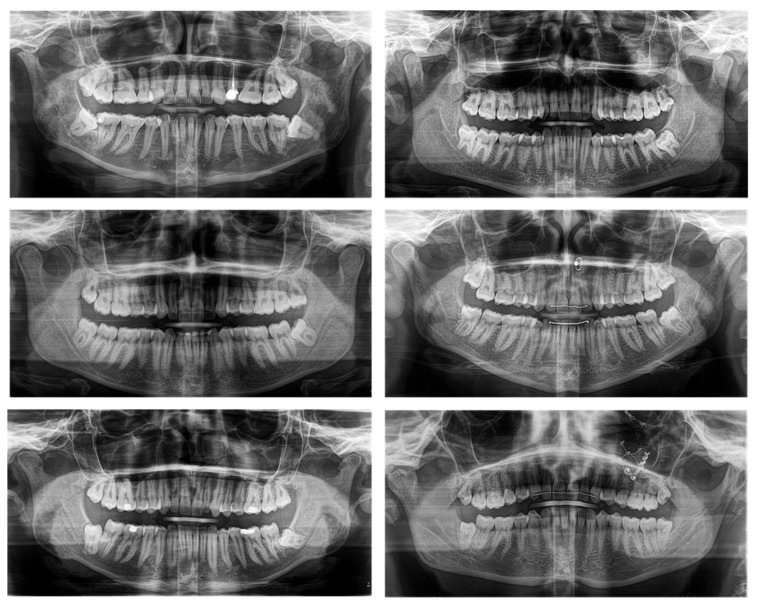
Study participants’ two-dimensional panoramic radiography (PAN) providing anatomical information about the angulation and position of the mandibular third molars (MTM) and the spatial proximity between the roots of the MTMs and the mandibular canal (MC). All participants showed an indication for the removal of MTMs and a close relationship between the MC and the MTM; therefore, these were considered high-risk cases and show indication for three-dimensional imaging.
